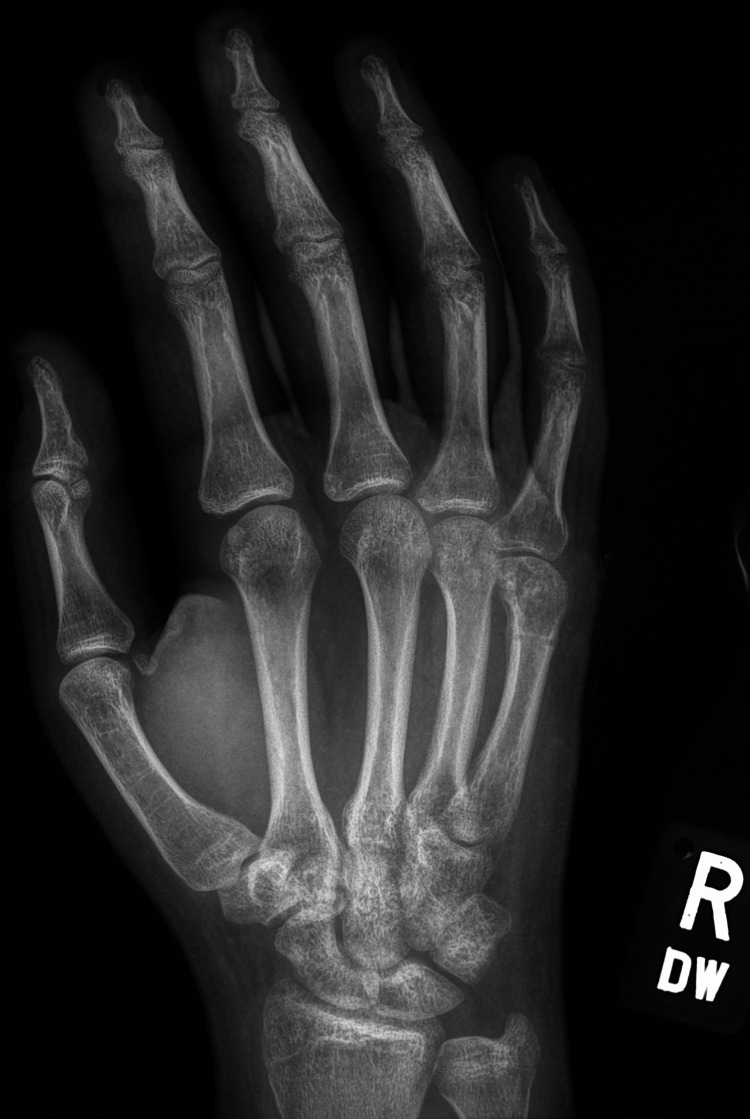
The patient's posteroanterior radiograph immediately following removal of the fixator.
